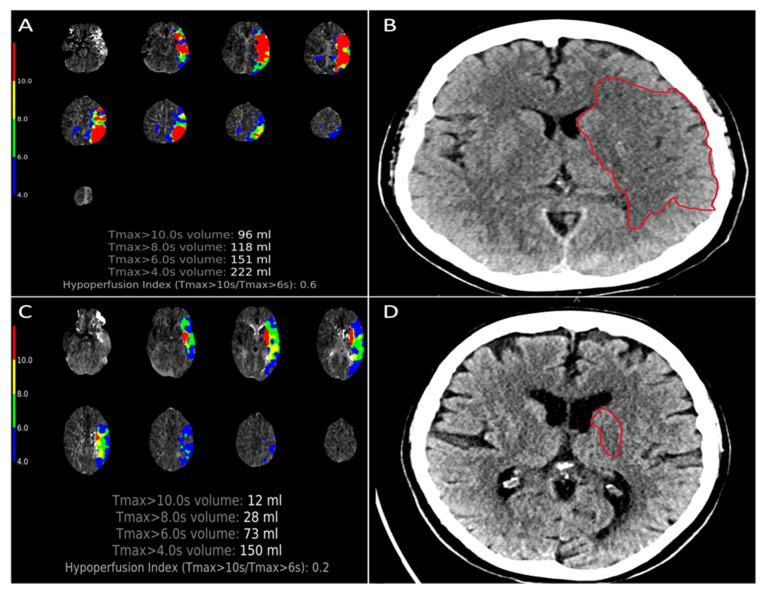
Imaging example of early edema progression in two patients with poor and favorable HIR. Two patients with comparable large vessel occlusion (proximal M1 occlusion) were both treated by endovascular thrombectomy. A 67-year old male patient (A,B) with symptom onset 2 h before admission imaging showing unfavorable HIR profiles (HIR = 0.6) and a considerable large baseline infarct hypodensity on non-contrast head CT (marked by the red field in (B)). The early edema progression rate was calculated at 5.18%/hour for this patient. A 71-year old female patient (C,D) who was last seen well 3.2 h before admission imaging exhibited only small infarct hypoattenuation in the basal ganglia (marked by the red circle in (D)), showing a favorable HIR profiles of 0.2 on admission perfusion imaging. The related early edema progression rate for this patient was calculated at 0.48% per hour.
